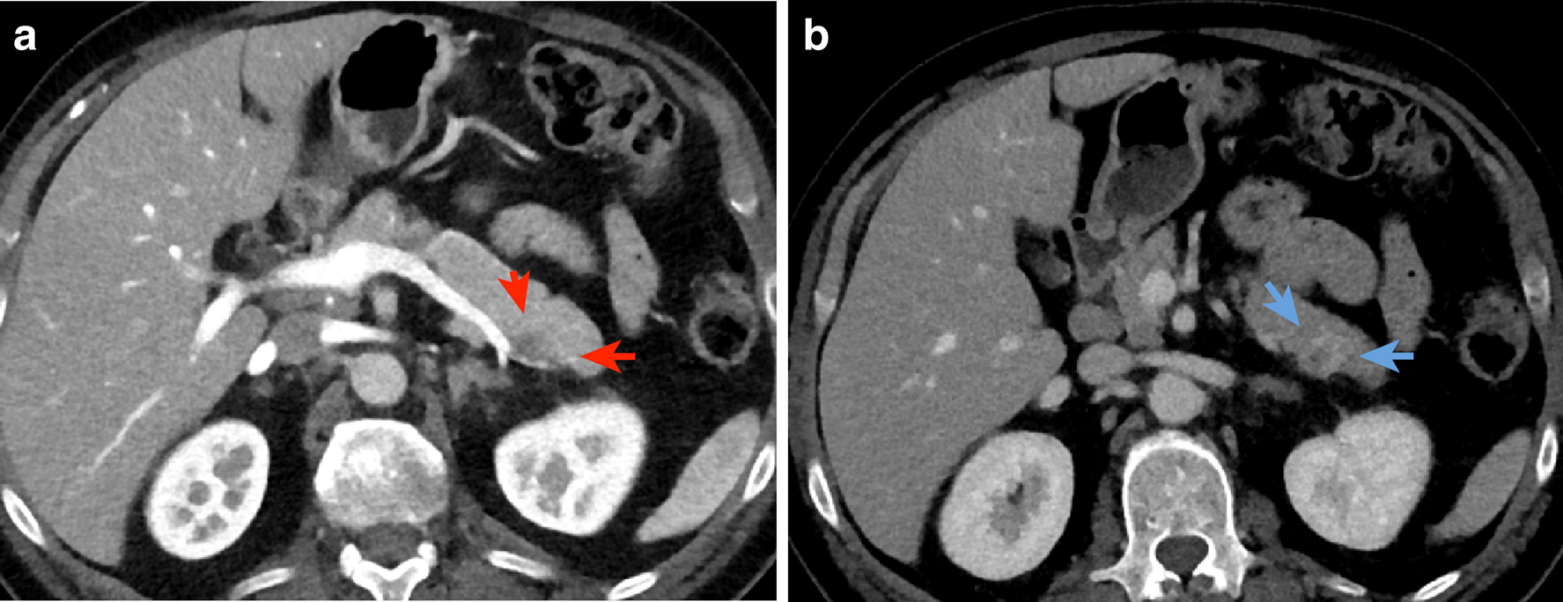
a CT of the pancreas with intravenous contrast in the arterial phase (*red arrows*) shows a 17 × 15-mm tumor with heterogeneous contrast enhancement. b CT of the pancreas in the venous phase shows the tumor (*blue arrows*) in the pancreatic tail
